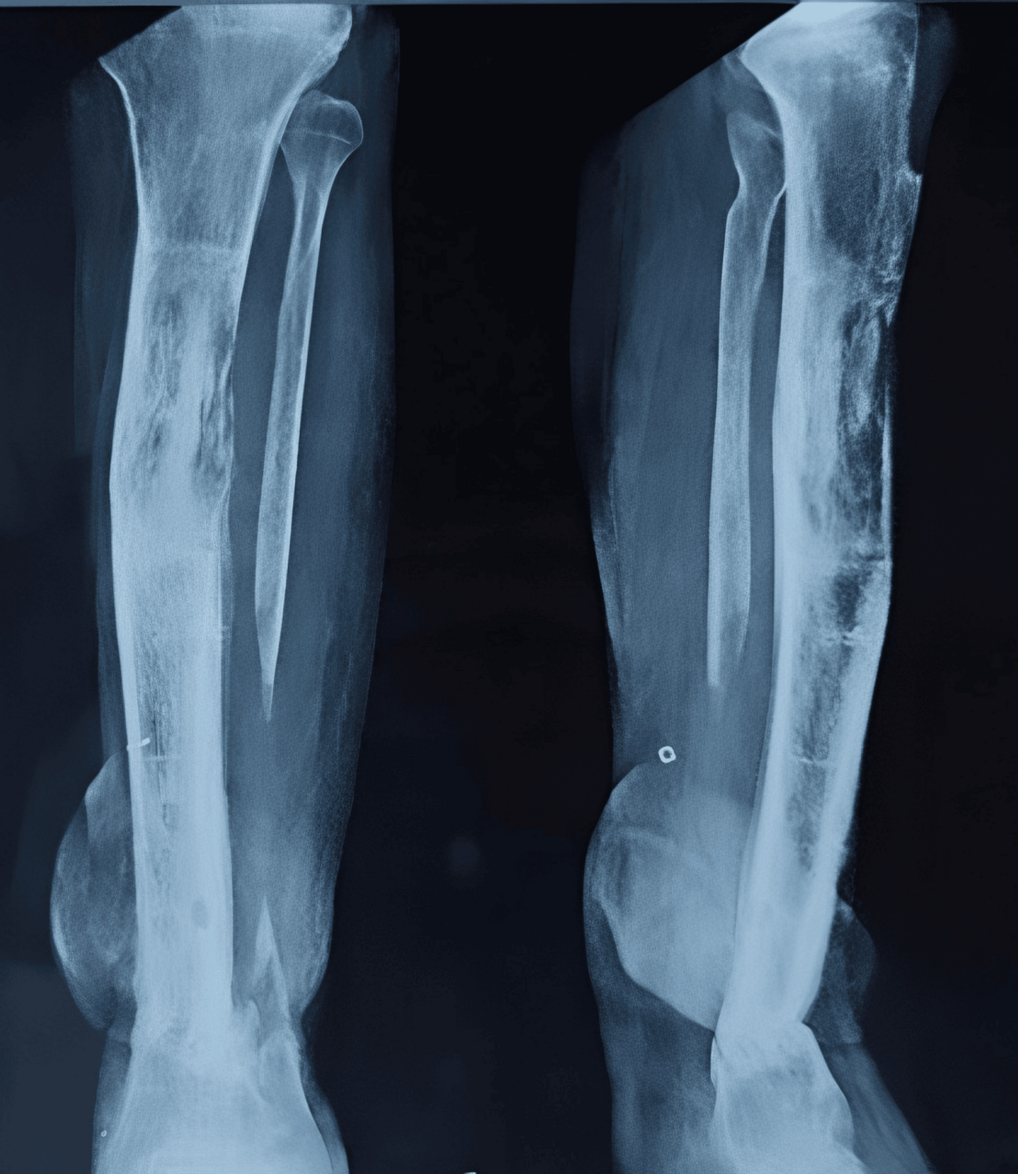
X-ray of the knee with the leg showing the complete healing at the nonunion site and consolidation at the distraction site
